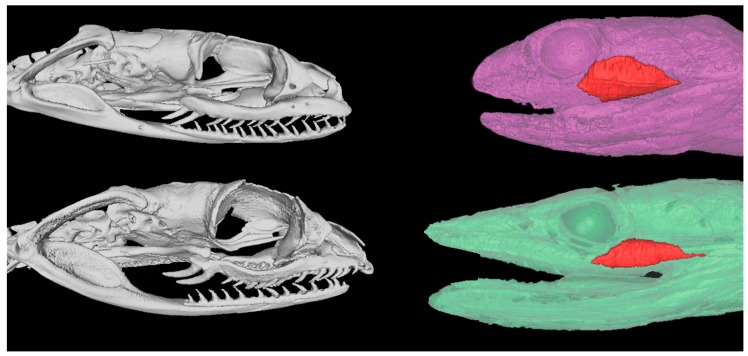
Contrast tomography (left column) and magnetic resonance imaging (right column) comparison of *D. typus* (top row) and *T. mossambicanus* (bottom row) venom delivery systems.
